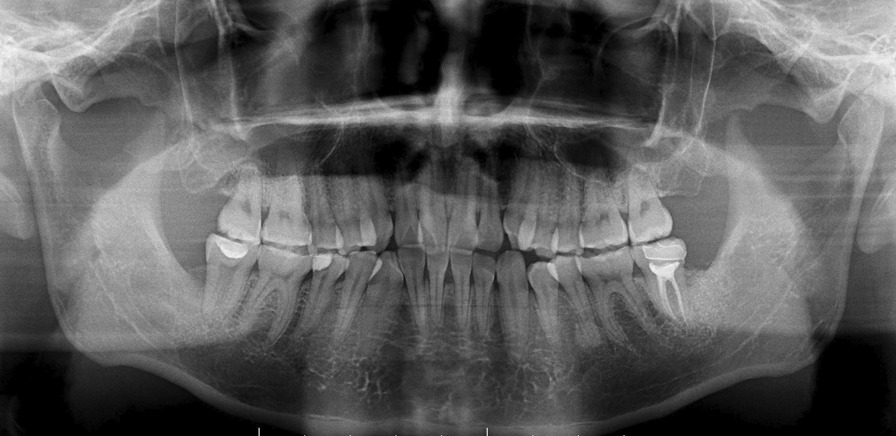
Postoperative panoramic radiographs
